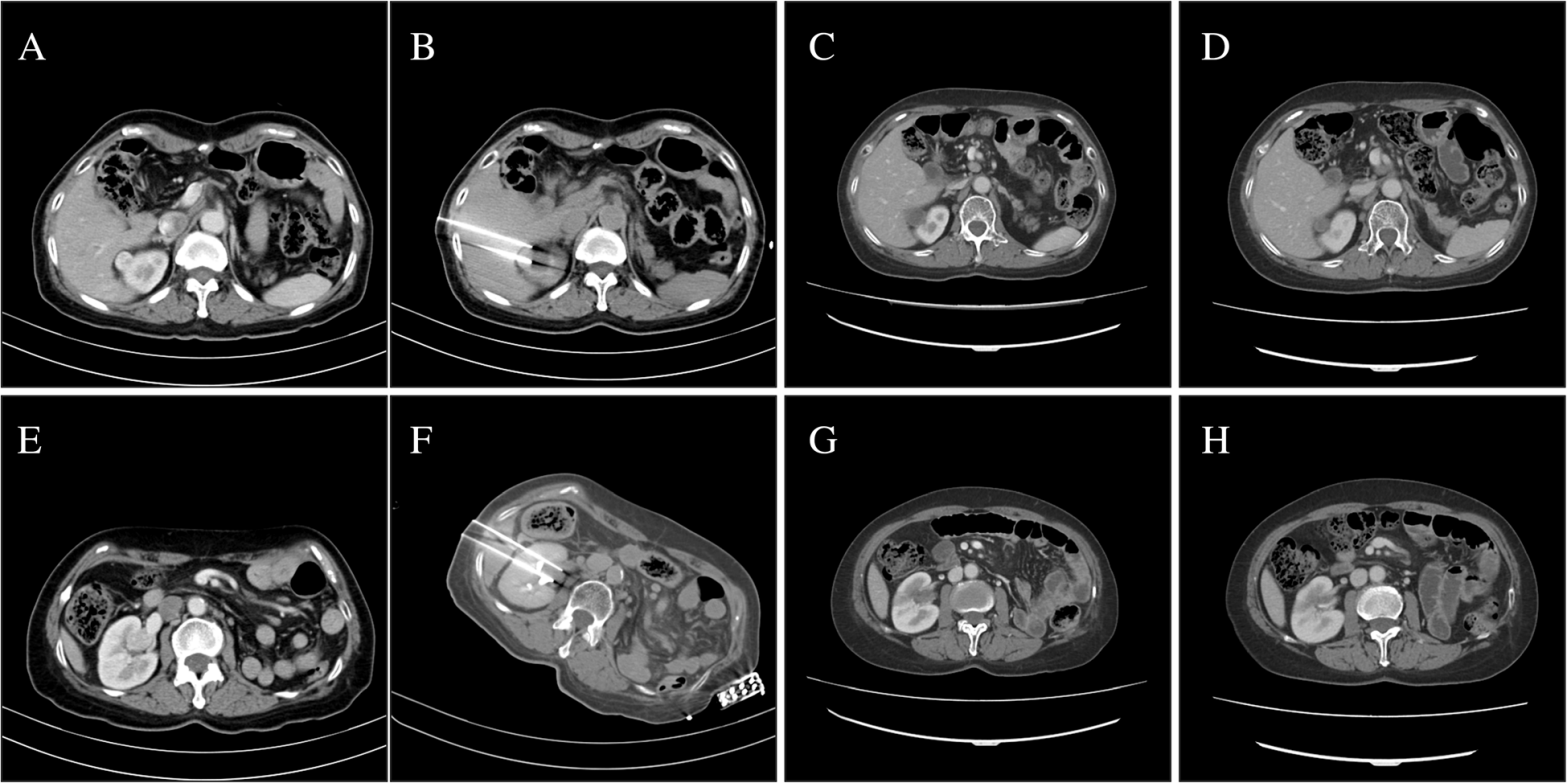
Solitary kidney patient with two RCC lesions. a-d The tumor located in the upper pole of the right kidney with the needle transhepatic approach showed no contrast enhancement 1 month (c) and 3 months (d) after IRE. e-h The tumor was adjacent to the renal pelvis with the needle transhepatic approach. Contrast-enhanced CT images obtained 1 month (g) and 3 months (h) after IRE demonstrated no enhancement in a well-circumscribed healing area without damage to the renal pelvis or large hilar vessels
